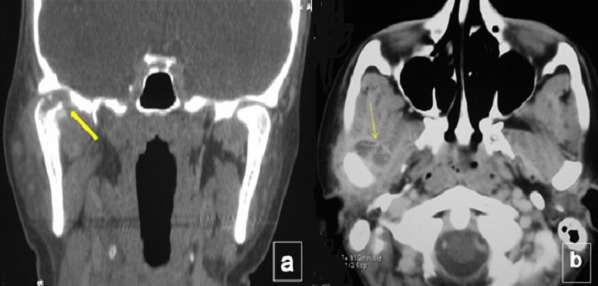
A) CT scan revealing osteolysis of the right mandibular condyle; B) two abscesses in the right infratemporal fossa
